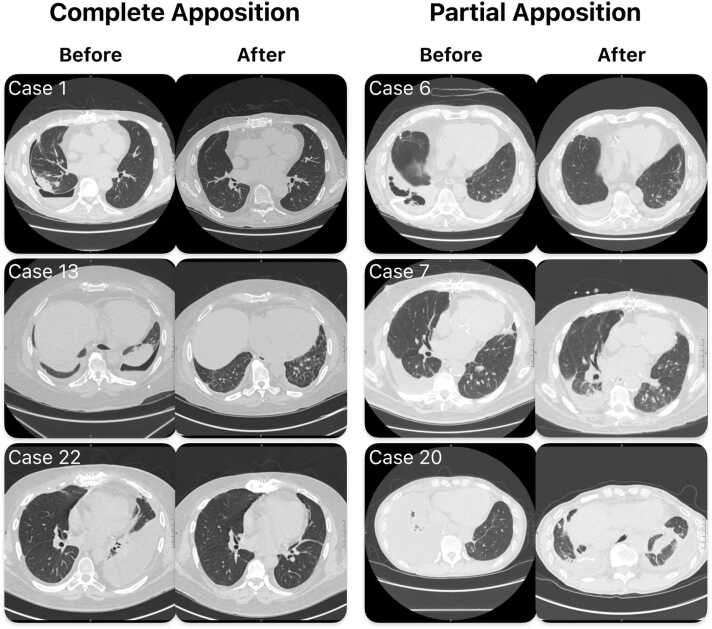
Lung apposition before and after robotic decortication. CT axial views in lung window showing representative cases showing before and 1 year after robotic lung decortication
